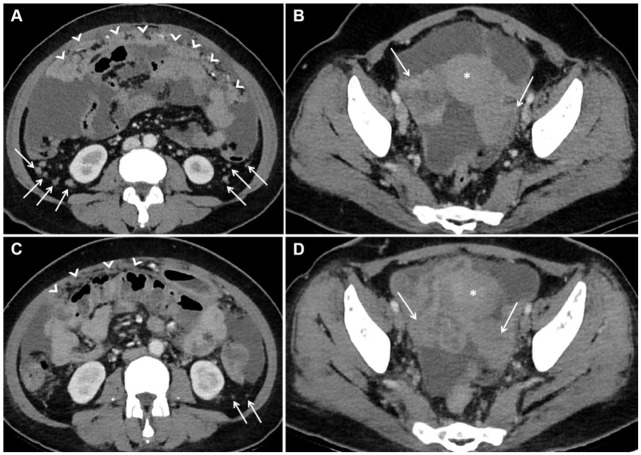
Case 6: Axial contrast-enhanced CT images before and after chemotherapy. (A) Before treatment, omental cake (arrowheads) and extensive peritoneal and retroperitoneal nodules (arrows) are visible. (B) Before treatment, a right cystic-solid ovarian mass and a left solid ovarian mass (arrows) are present; the uterus is marked by an asterisk. (C) After 4 cycles of chemotherapy, the omental cake has markedly regressed (arrowheads) and the retroperitoneal nodules have nearly disappeared (arrows). (D) After 4 cycles of chemotherapy, the right cystic-solid ovarian mass has significantly reduced in size, the left solid ovarian mass has mildly reduced (arrows), and the uterus remains visible (asterisk).
